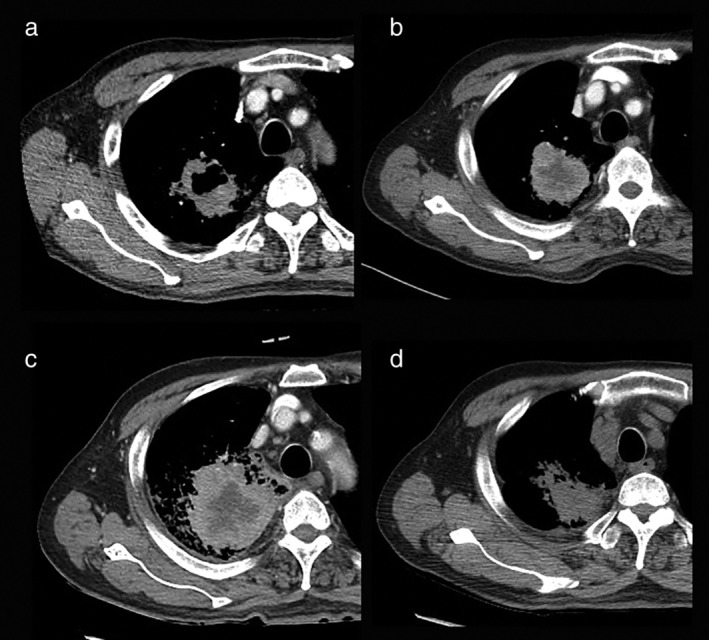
Representative chest computed tomography images (a) at diagnosis, (b) before administration of nivolumab, (c) after the first dose and (d) after the third dose of nivolumab treatment when the size of the lung mass and the ground‐glass opacities around the mass had decreased dramatically.
